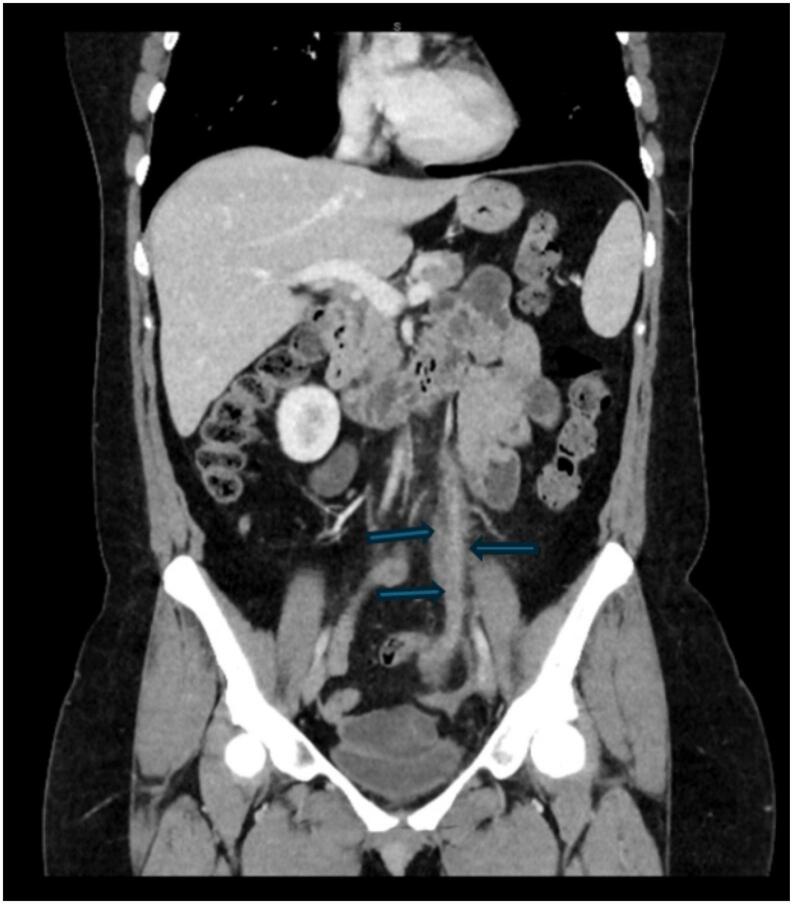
Coronal reconstructed contrast-enhanced CT image demonstrating distended poorly enhancing left ovarian vein (arrow) with subtle surrounding inflammatory reaction. The distal most segment of the left ovarian vein is normal calibre and enhances.
